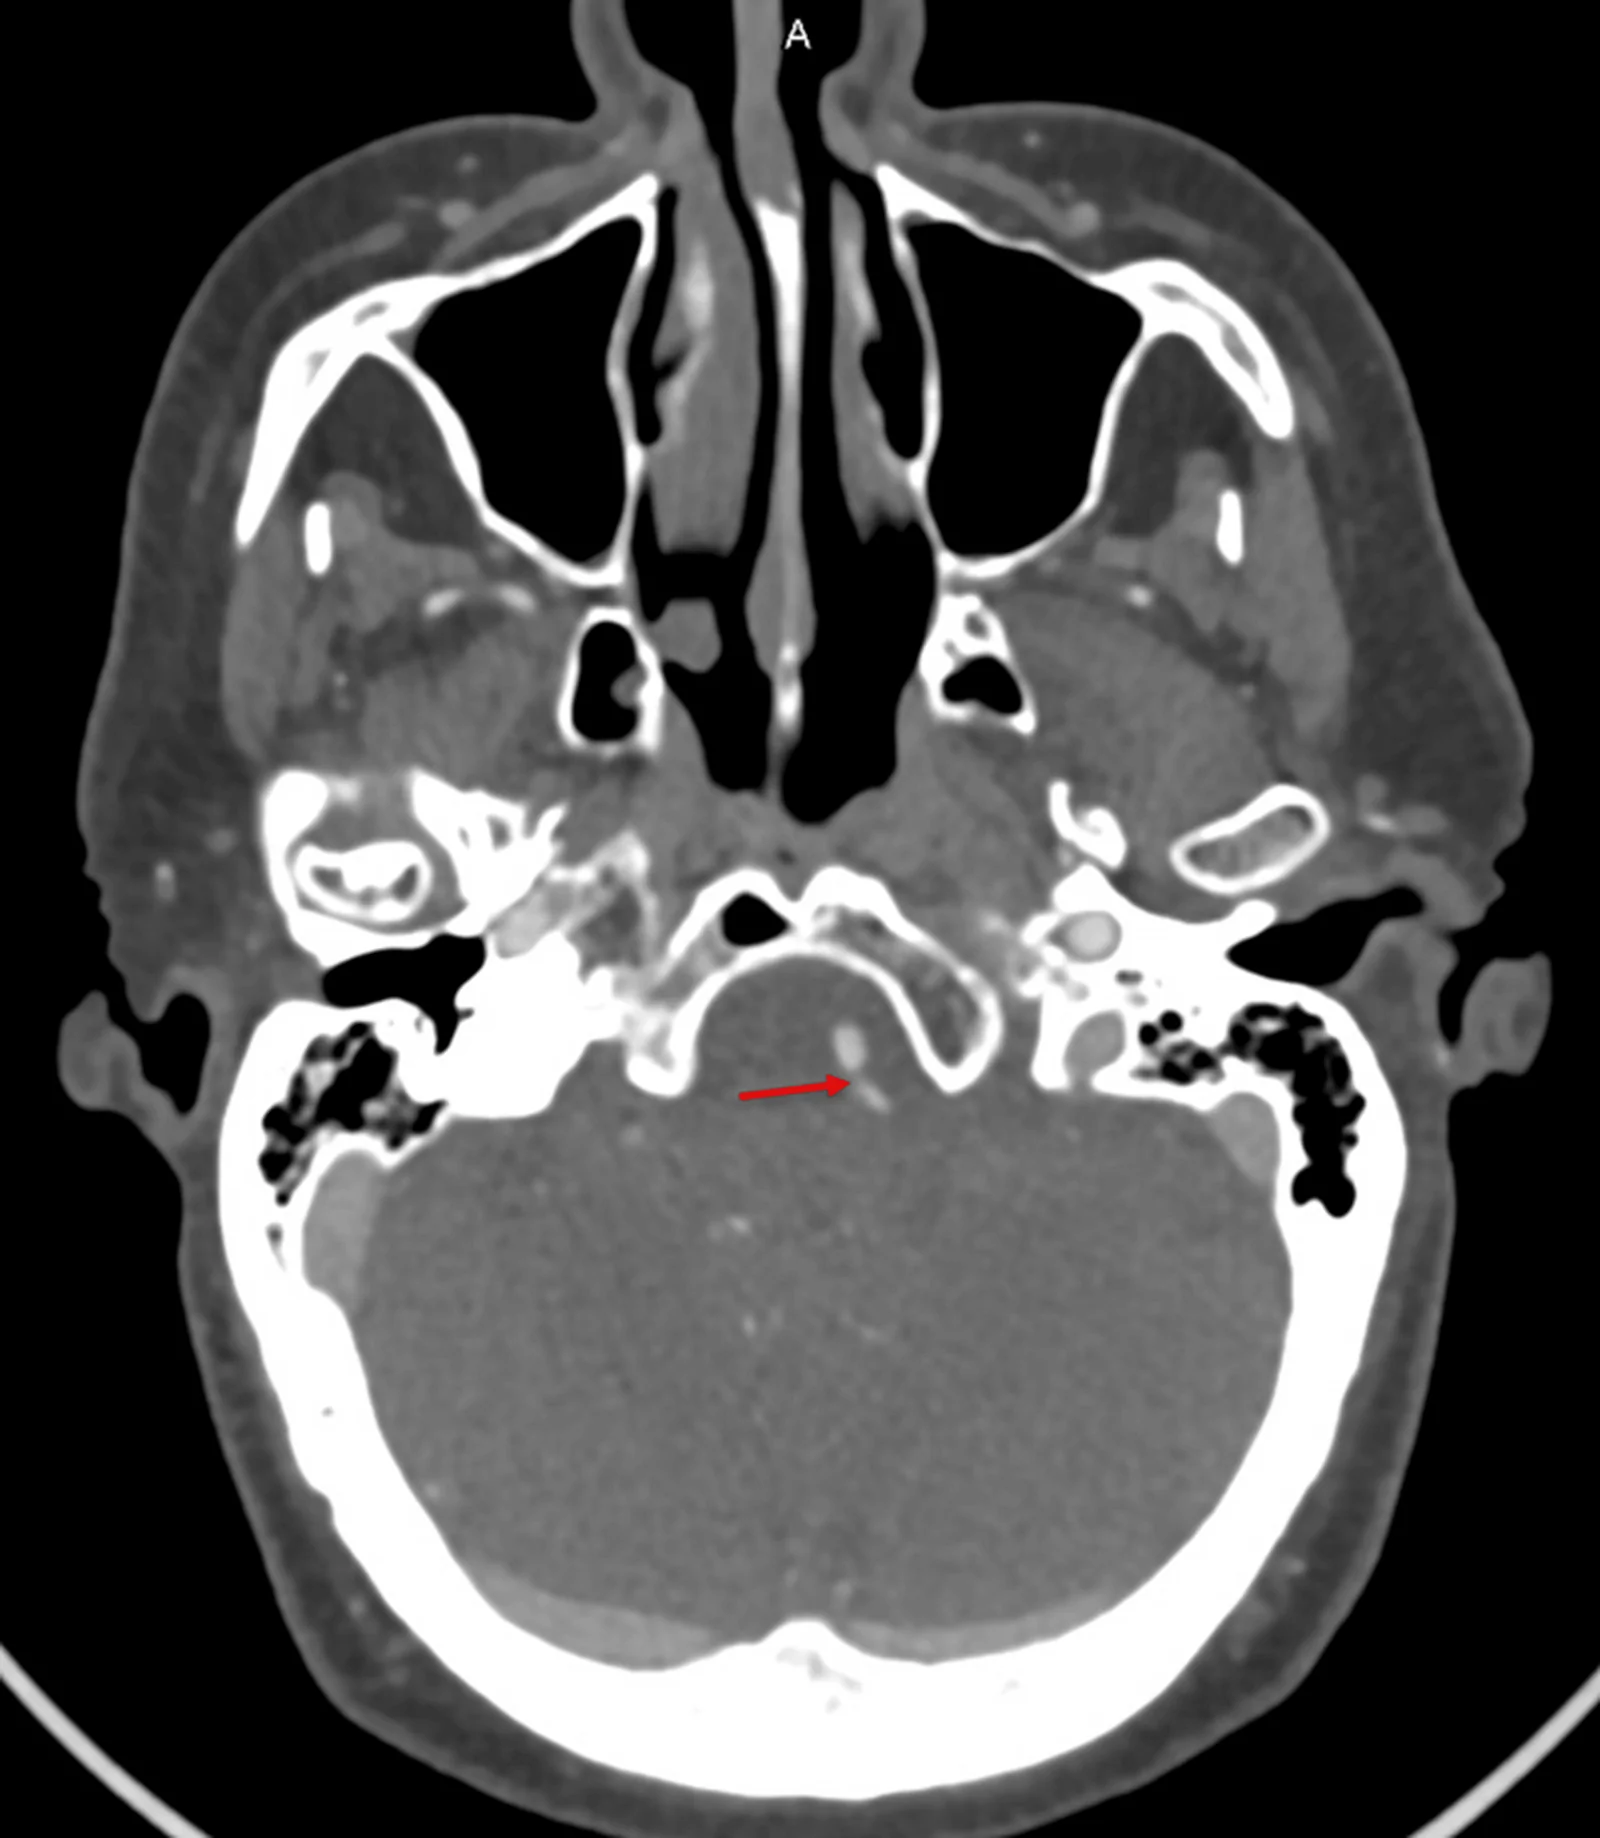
Computed tomography angiography of the supra-aortic and intracranial vessels showing subocclusive stenosis of the left V4 vertebral artery segment (arrow)
